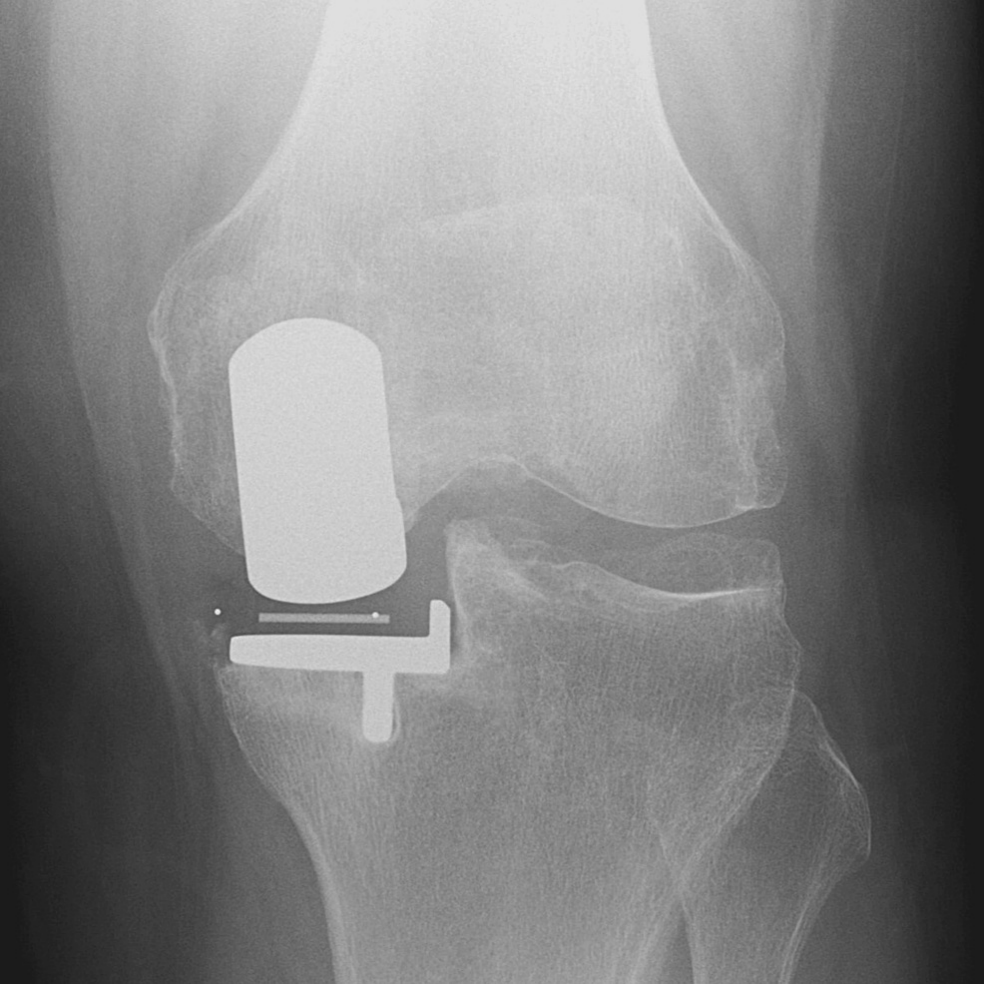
Radiography at three months postoperatively.
